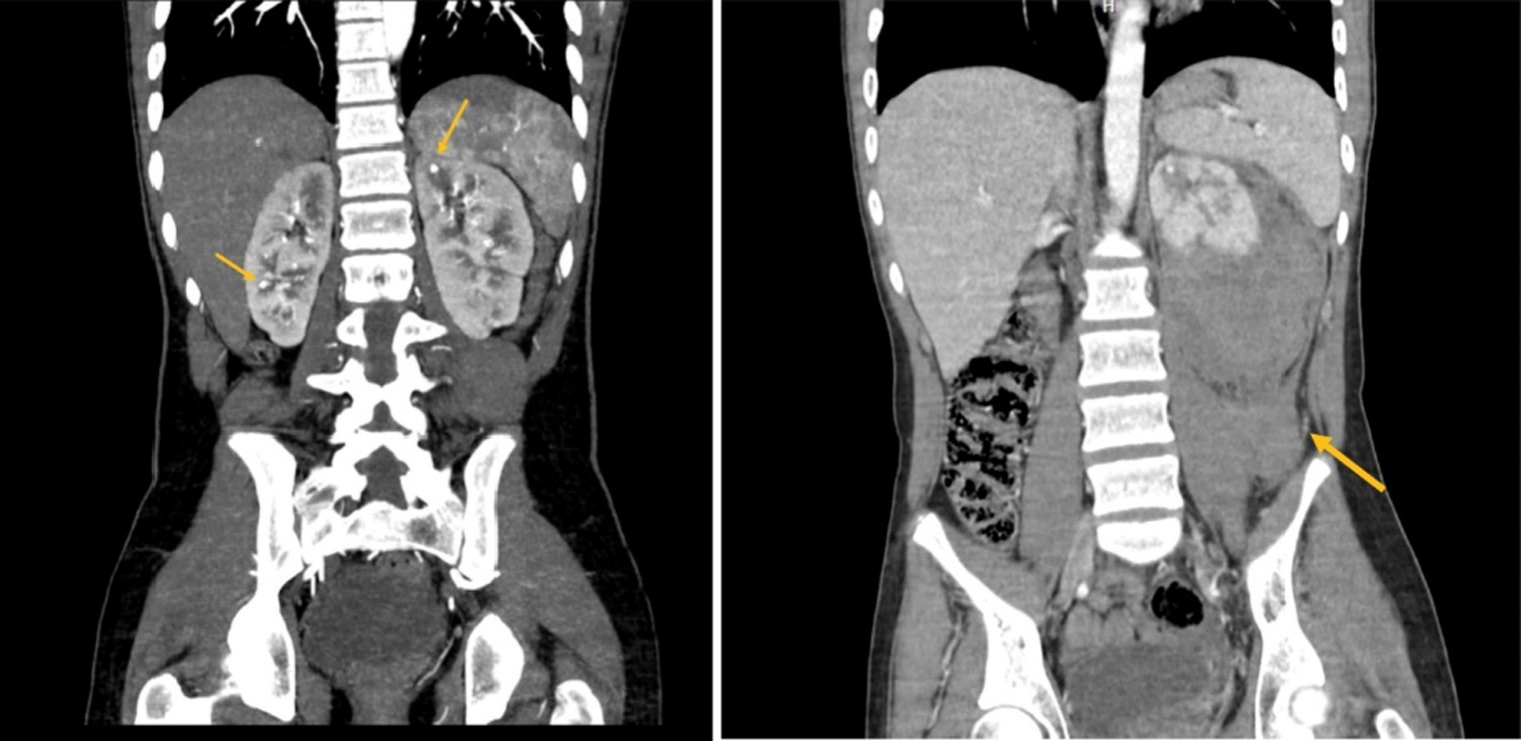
Coronal CT scan of abdomen with previous infarct.
